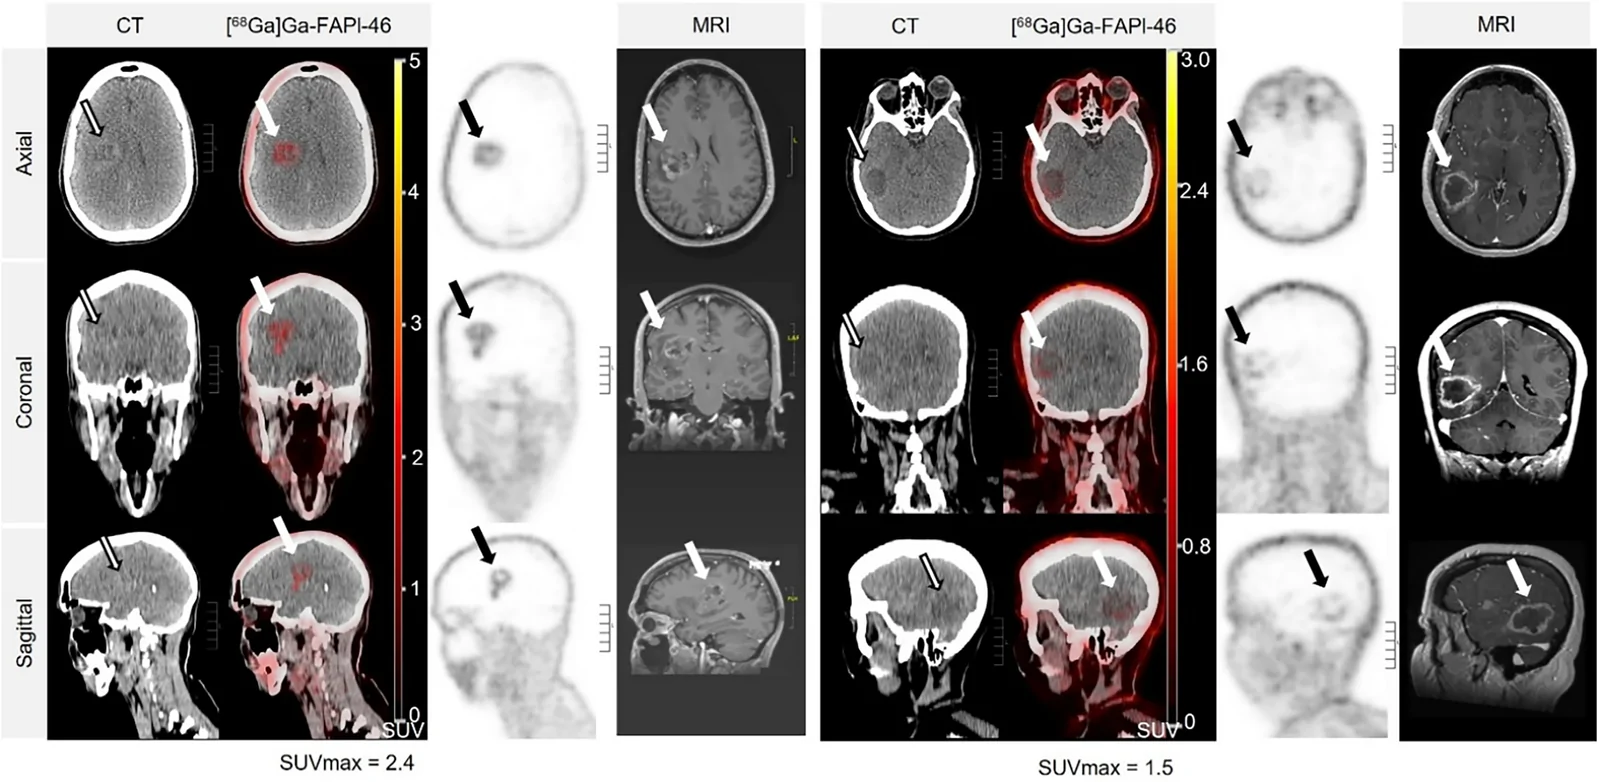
[^68^Ga]Ga-FAPI-46 PET/CT images of patients. [^68^Ga]Ga-FAPI-46 PET/CT scans were performed for 2 patients. SUV_max_ was 2.4 and 1.5. PET/CT images are shown alongside corresponding MR images. Arrows indicate location of tumor.
